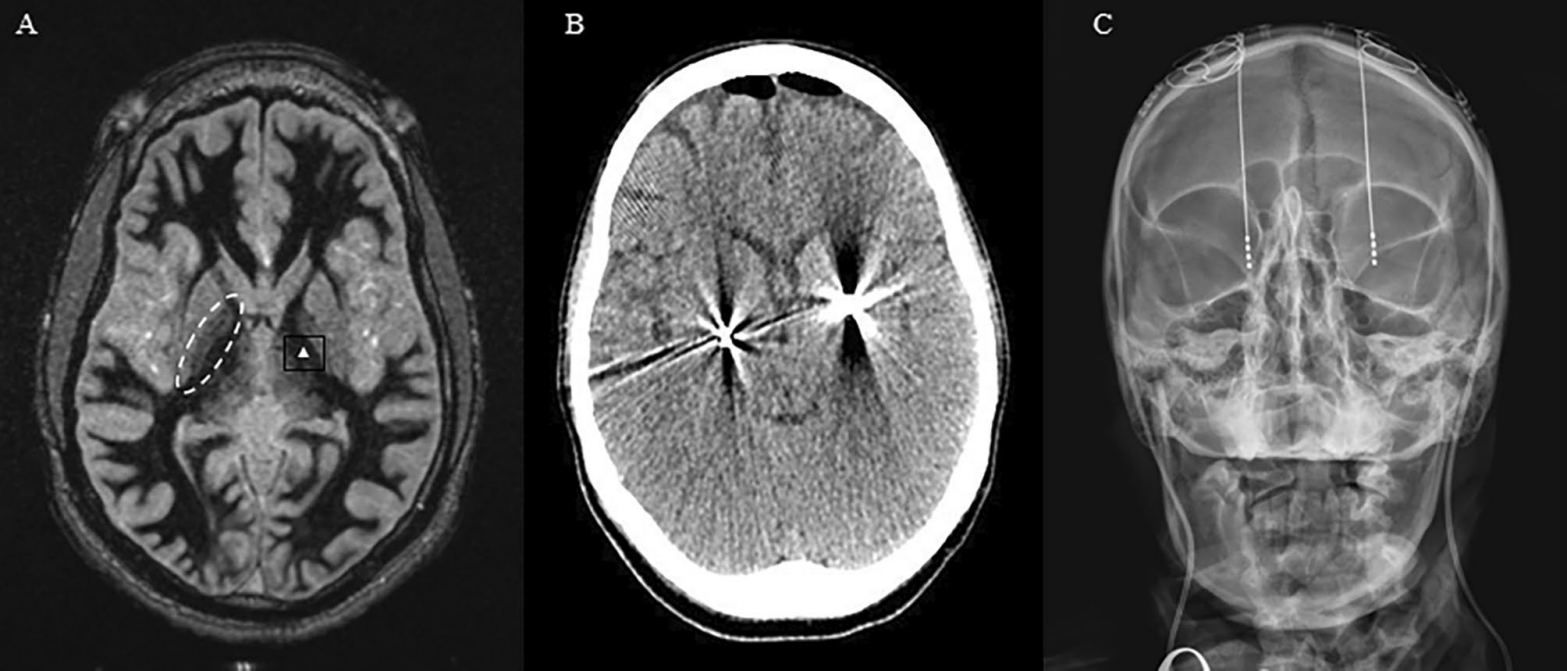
A FGATIR MRI sequence used for direct visualization of the GPi (dashed oval). The GPi target is marked with a white triangle. The position was determined using the technique described by Starr and colleagues [76]. Placement of bilateral leads into GPi on postoperative B CT scan and C x-ray
